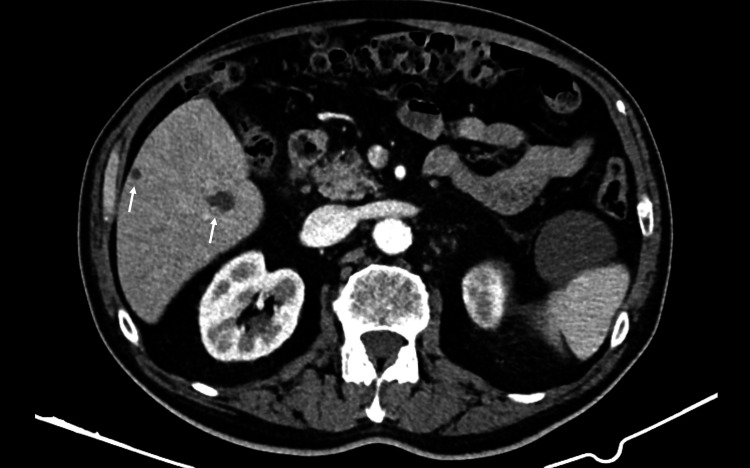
Abdominal CT scan showing hepatic nodules (arrows)
